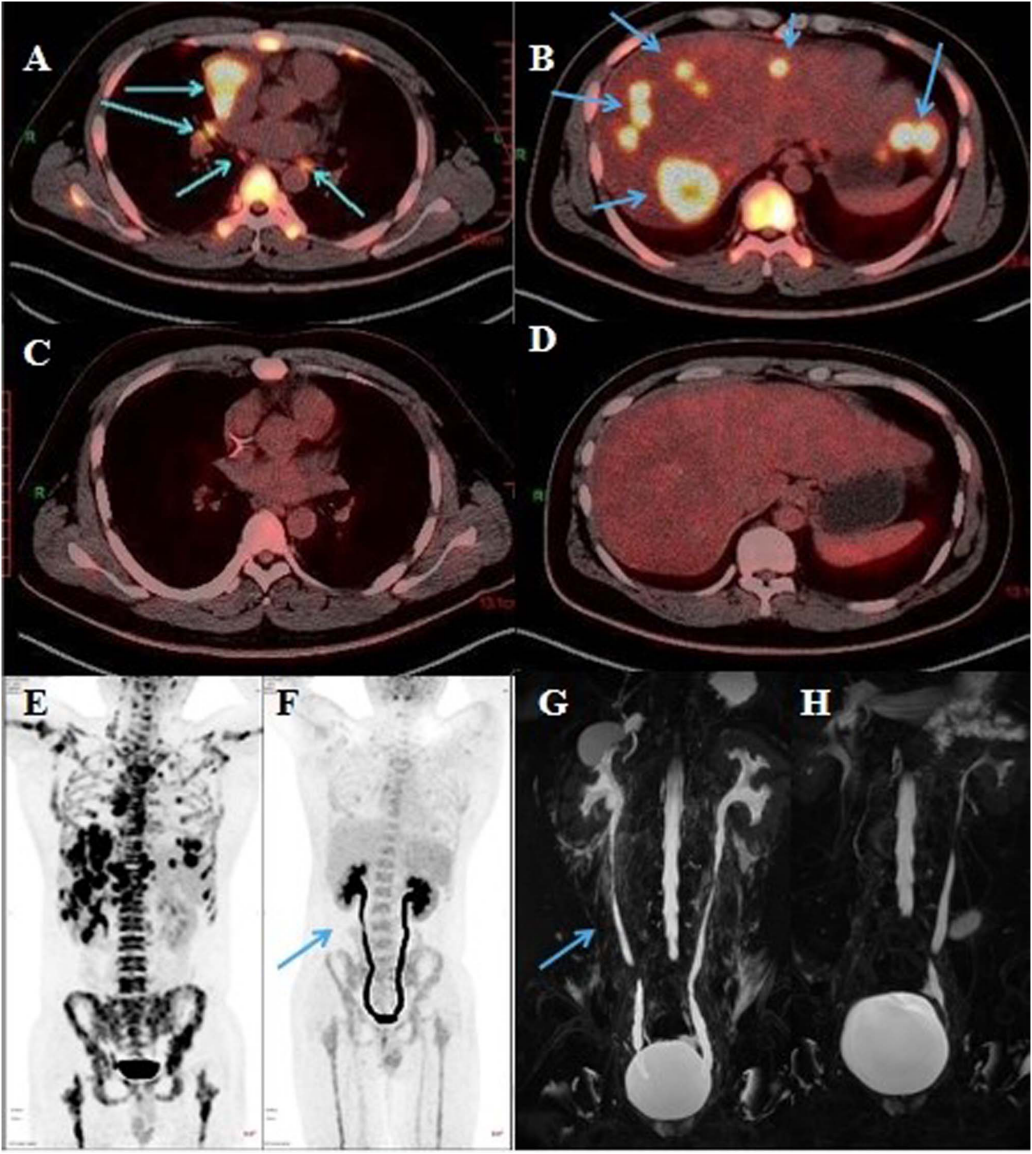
Representative radiological images of the patient. (A) Mediastinum tumor and (B) hepatic metastatic lesion on PET/CT (April 11, 2022). (C) Mediastinum tumor and (D) hepatic metastatic lesion on PET/CT (August 24, 2022). (E) PET/CT (April 11, 2022). (F) Bilateral ureters were slightly dilated on PET/CT (blue arrow, August 24, 2022). (G) Bilateral ureteral wall was slightly thickened and whole ureteral was full expanded on urinary MRU (blue arrow, August 31, 2022. (H) Right ureter expansion was improved, and ureteral exudation was absorbed on urinary MRU (September 22, 2022).
